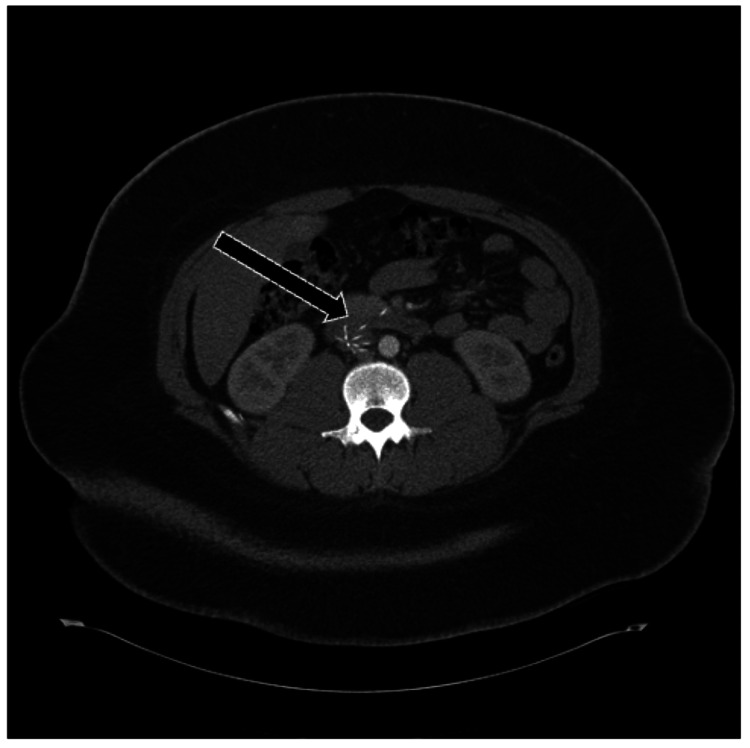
IVC Filter Complication: Strut Perforation Into the Duodenum Axial non-contrast CT of the abdomen demonstrating an infrarenal IVC filter complication. One of the filter struts is displaced, perforating the wall of the third portion of the duodenum (arrow). Recognition of adjacent organ perforation is critical, as it can lead to hemorrhage, infection, or perforation-related sequelae requiring urgent intervention.
